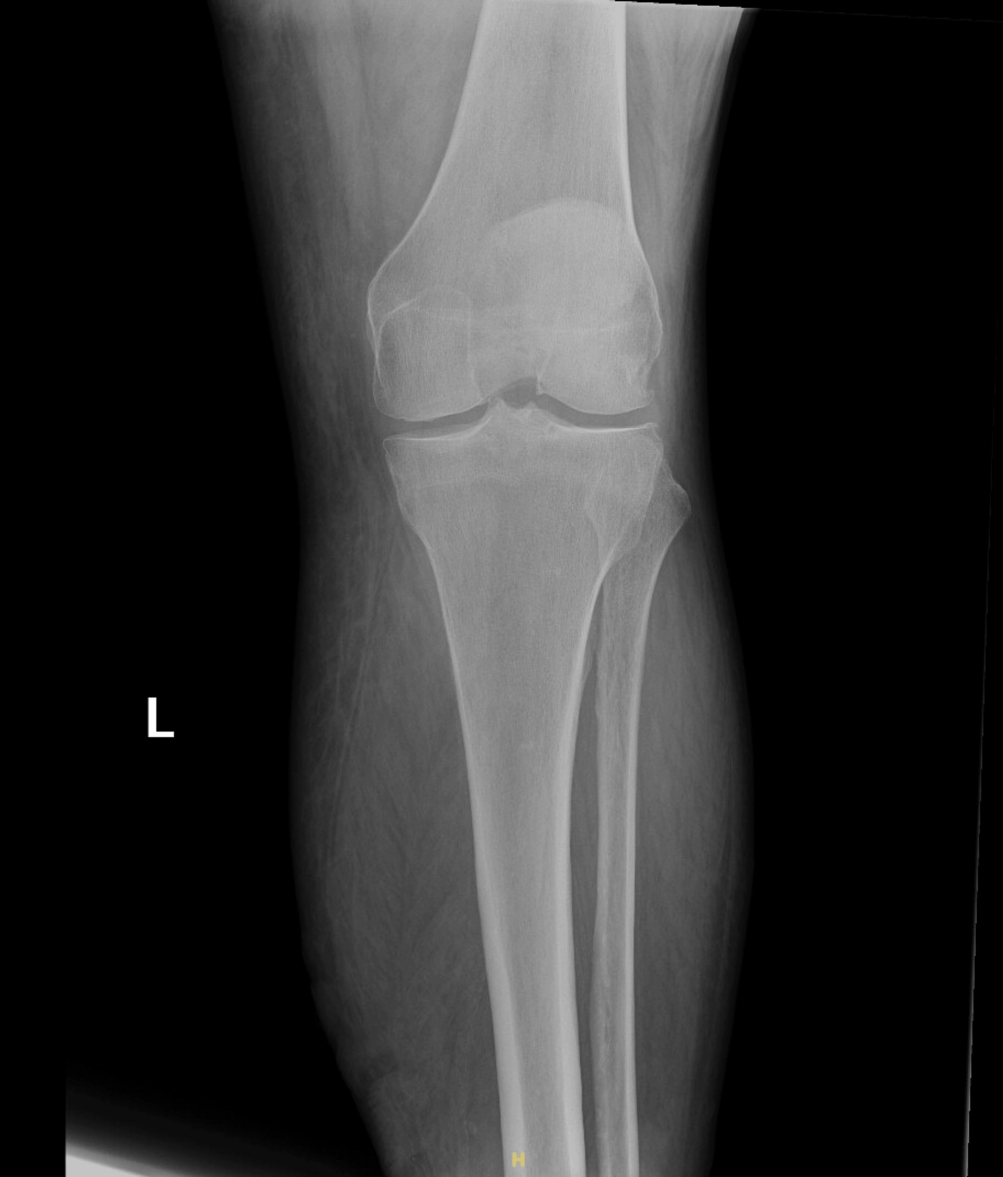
X-ray of the left tibula and fibula shwoing edematous and irregular soft tissues at the left leg and ankle.
